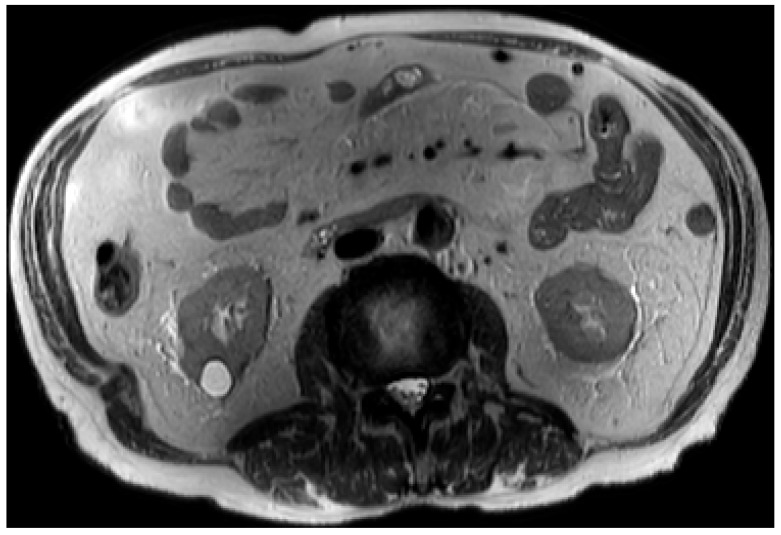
Axial T2-weighted MRI scan demonstrating a low-signal-intensity pseudocapsule, displacement of adjacent bowel loops, embedded nodules, a fat ring sign around mesenteric vessels and nodules, and reduced mesenteric signal intensity, consistent with MP.
